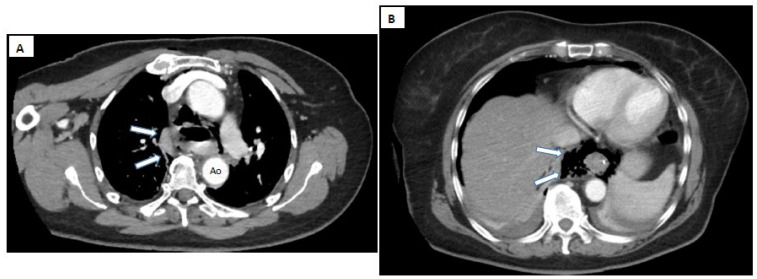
CT scan of the chest (A) and upper abdomen (B) with contrast agent and cross-sections. Images of a significant hydro-air collection at the mediastinal level (arrows), with extension into the upper abdomen through the esophageal hiatus through an esophageal rupture. Ao—aorta.
